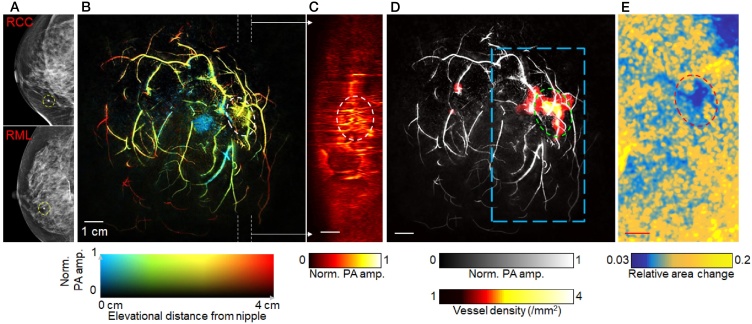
From the Caltech Single-Breath-Hold ring-shaped imager: (A) X-ray mammograms of a 69-year-old female patient with an invasive ductal carcinoma (grade 2/3). RCC, right cranial-caudal; RML, right medio-lateral. (B) Depth-encoded PA image. (C) Maximum amplitude projection (MAP) images of thick slices in sagittal plane. (D) Automatic tumor detection on vessel density maps. (E) Maps of the relative area change during breathing. (Reproduced with permission of the authors and publisher.)
